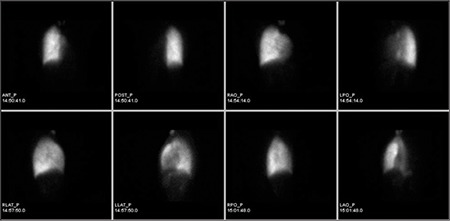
Anteroposterior, left and right lateral, left and right posterior oblique, left and right anterior oblique images of Tc-99m macroaggregated albumin (MAA) perfusion scintigraphy shows absent left lung perfusion
